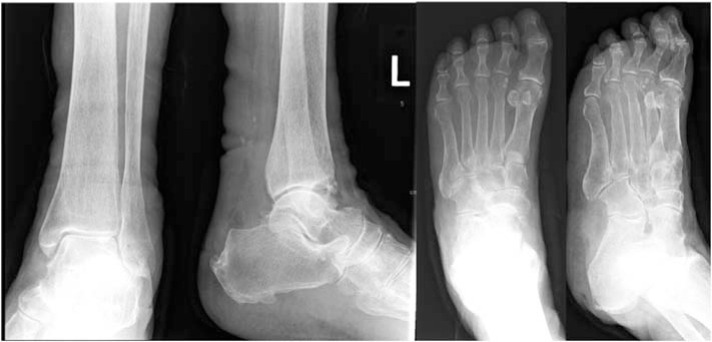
Radiographs of a patient who presented with multiple foot abscesses, showing osteopenic bones without erosive changes.
